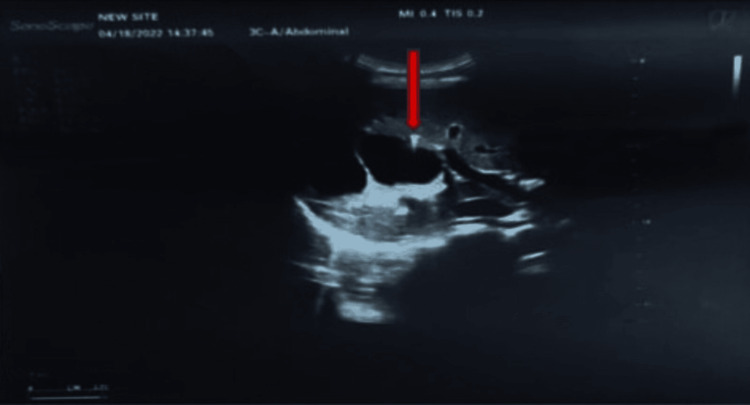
Abdominal ultrasound Hyperechoic foci, with comet tail artifacts (red arrow) and hydatid cyst between segments VI and VII measuring 80.5 x 68 x 60 mm, classified as CE2 according to WHO classification
